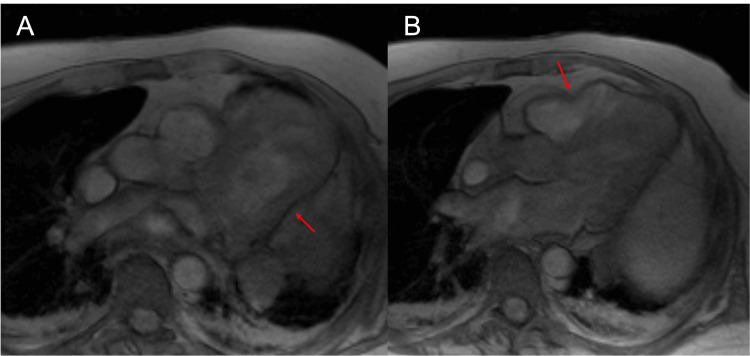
Cardiac magnetic resonance imaging, axial gradient echo (GRE) sequence Pericardial defect with herniation of both ventricles. Notch in the contour of the ventricular margins at the level of the pericardial defect (Red arrows). There are no calcifications.
